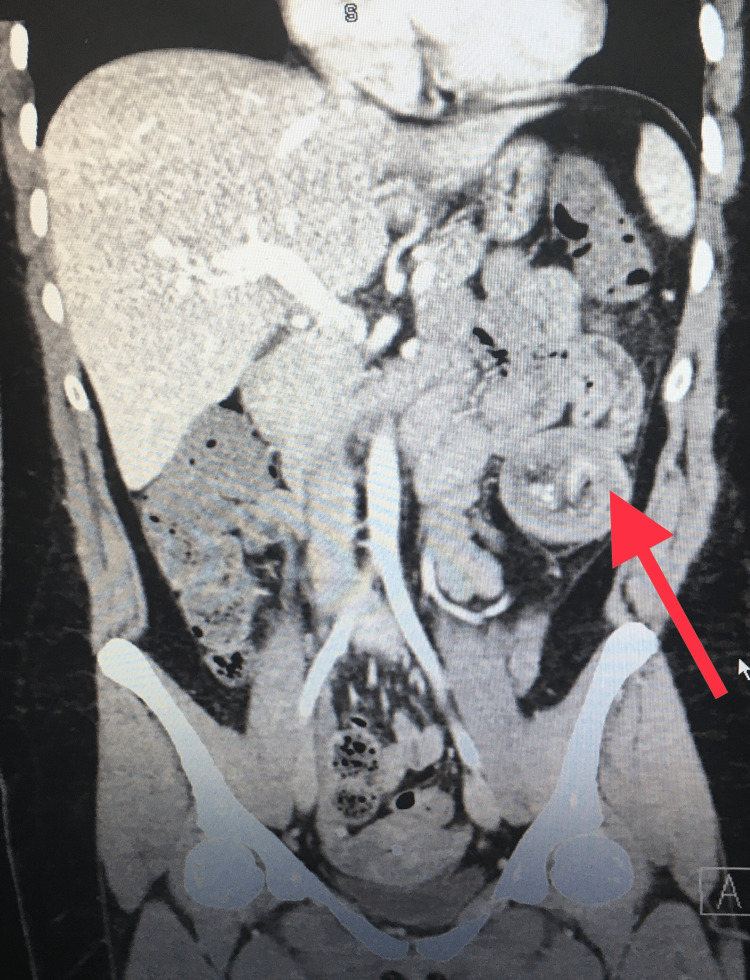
Coronal view of the CT scan of the abdomen and pelvis Red arrow: Target sign on the intussusception of the descending-sigmoid colon
